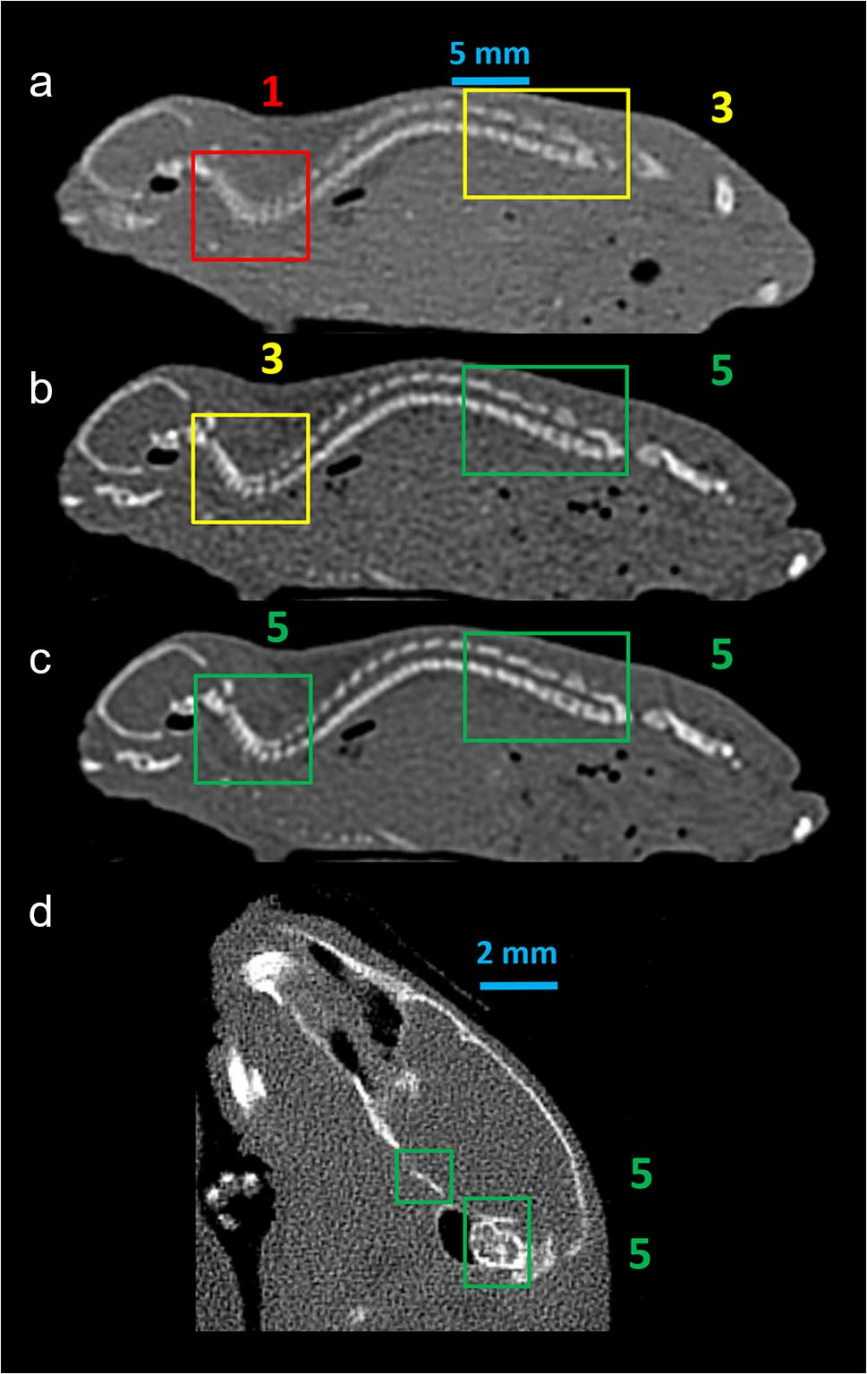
Semiquantitative analysis of bone detail visualization. Examples for semiquantitative analysis of bone detail visualization. 1 = no delineation, 2 = unsharp delineation, 3 = sharp delineation. a: EID-CT at CTDI_Vol_ = 5 mGy, b: PCD-CT at CTDI_Vol_ = 3 mGy, c: PCD-CT at CTDI_Vol_ = 10 mGy. CTDI_Vol_, volumetric CT dose index; EID-CT, energy-integrating detector CT; PCD-CT, photon-counting detector CT
